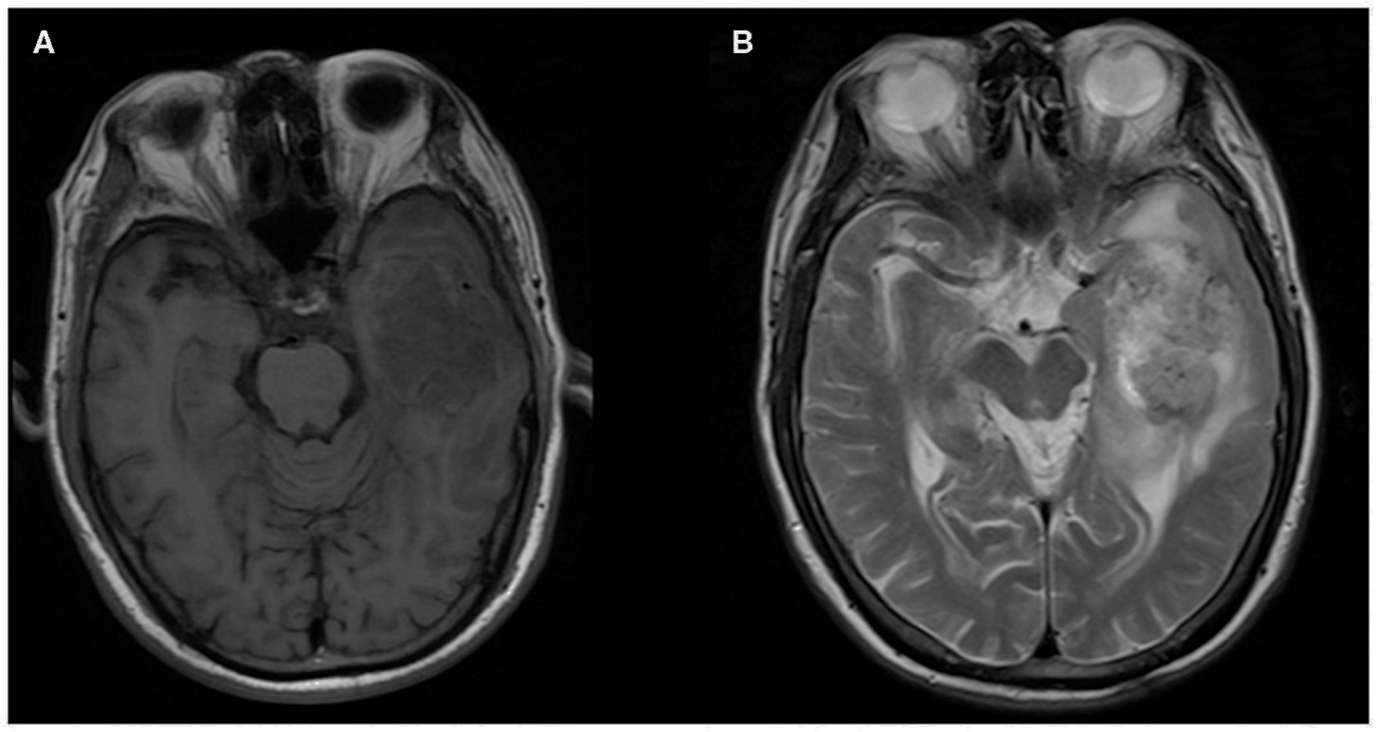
A 76-year-old man with supratentorial intraparenchymal anaplastic ependymoma in the left temporal lobe on (A) T1-weighted image and (B) T2-weighted image. The tumor appears as heterogeneous signal intensity and moderate peritumoral edema.
